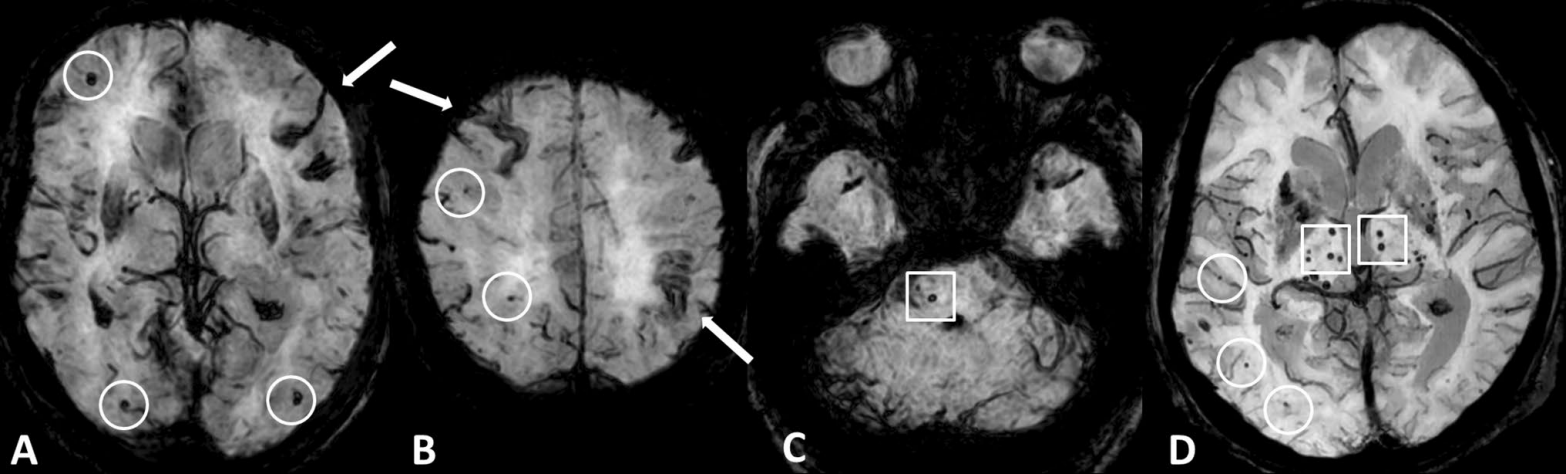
a, b Susceptibility-based MRI in a patient with probable CAA. Cerebral microbleeds in lobar location (circles) and cortical superficial siderosis (arrows). c, d Susceptibility-based MRI in a patient with mixed CMB. Cerebral microbleeds in lobar location (circles) and additional deep microbleeds (boxes) in the brain stem (c) and thalami (d)
